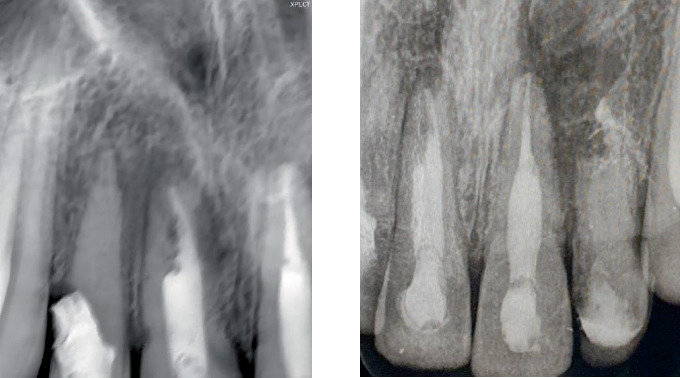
Intraoral periapical radiographs of 36 months' follow-up.
